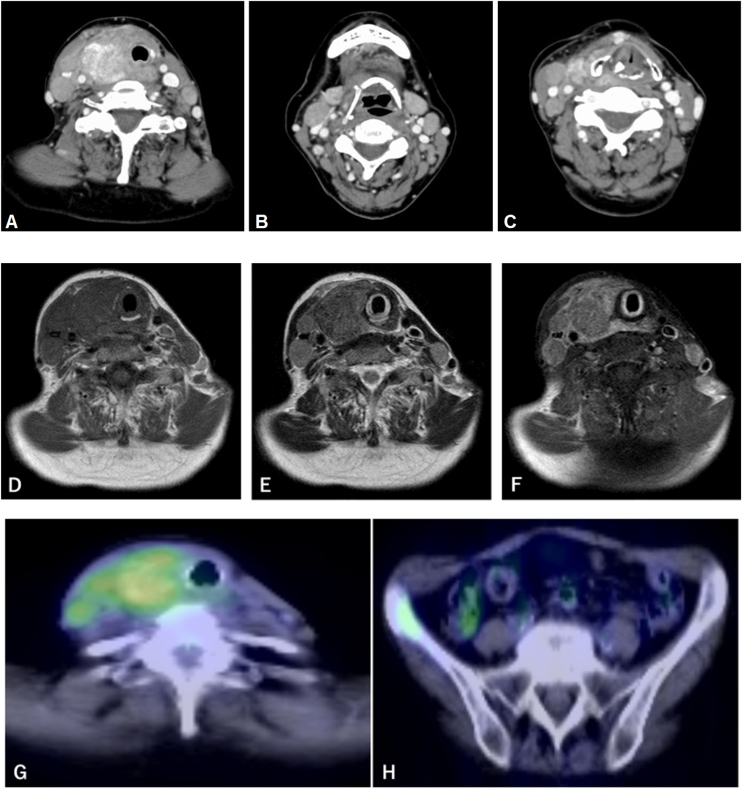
CT, MRI, and FDG-PET/CT images. (A–C) Contrast-enhanced Computed Tomography (CT) image showing a shadow with irregular margins and calcification in the right thyroid gland and swollen bilateral lymph nodes. (D‒F) Magnetic Resonance Imaging (MRI) showing a right thyroid tumor with low signal intensity on T1-weighted images (D), iso-signal intensity on T2-weighted images (E), and heterogeneous strong enhancement by gadolinium (F). (G‒H) Fluorodeoxyglucose Positron Emission Tomography/CT (FDG-PET/CT) image showing abnormal FDG accumulation in the right thyroid gland, bilateral cervical lymph nodes, and right iliac bone.
